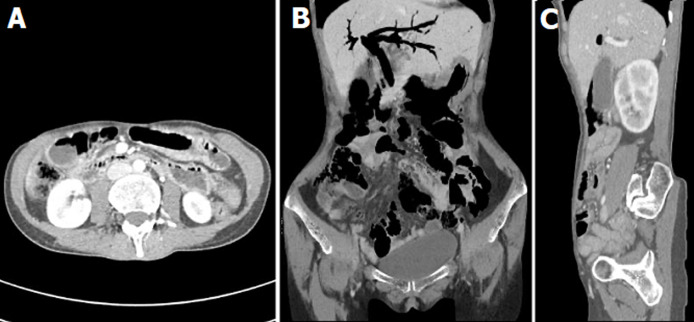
computed tomography findings: A) axial view; B) coronal view; C) sagittal view
